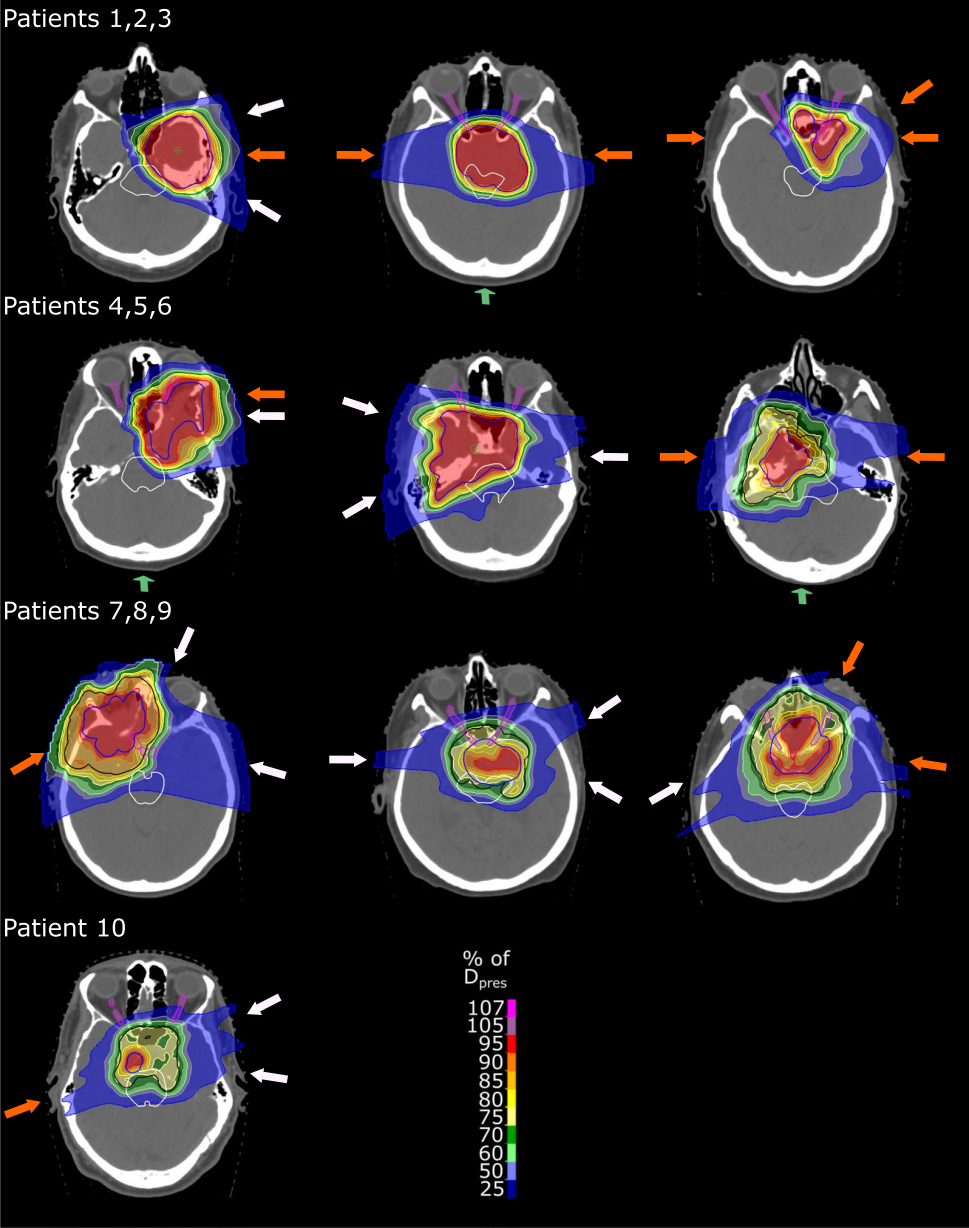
Constant relative biological effectiveness weighted dose distributions of the dose-only optimized reference plans in representative computed tomography slices. Isodoses are displayed relative to the prescription dose to the primary (high-dose) clinical target volume. Arrows depict coplanar (white) and non-coplanar beams (orange) as well as beams passing through the skullcap (green), respectively. Contours show the primary (high-dose) planning target volume (PTV, blue), secondary PTV (black), brainstem (white) and both optic nerves and chiasm (all magenta)
